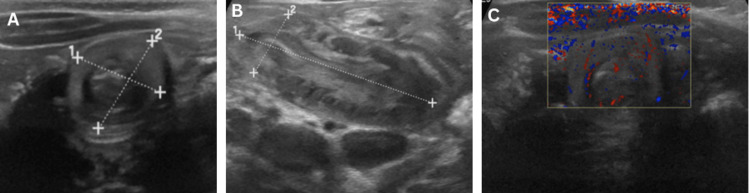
First episode of ileo-ileal intussusception. (A) Transverse ultrasound view demonstrating the classic target sign. (B) Longitudinal view showing pseudokidney appearance consistent with ileocolic intussusception. (C) Doppler image demonstrating preserved bowel vascularity.
